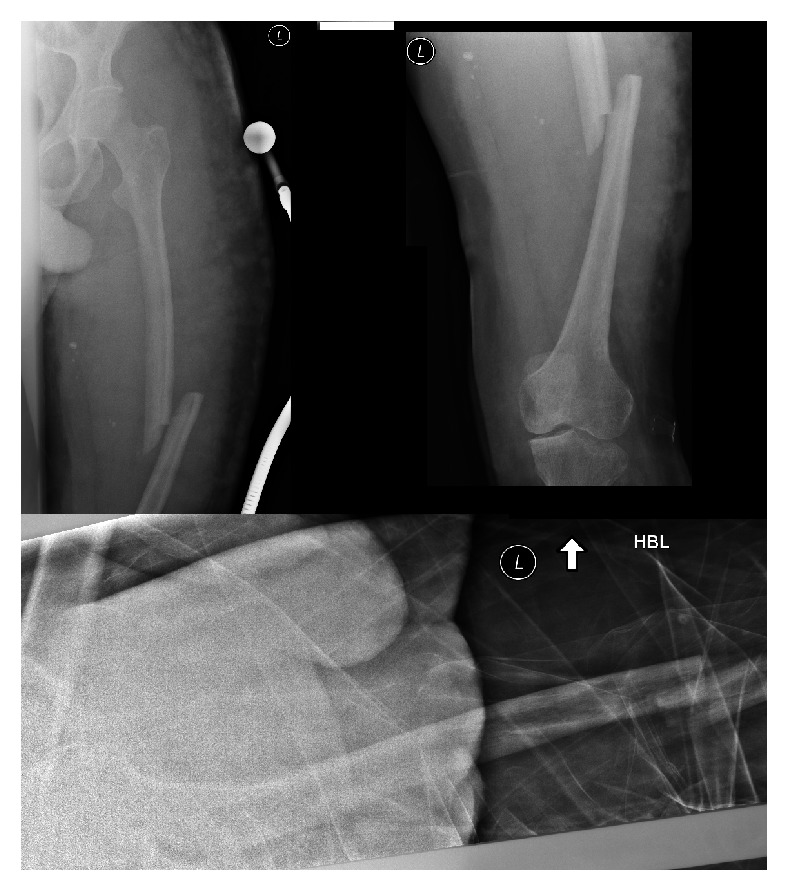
AP and lateral radiographs of the left femur, demonstrating a complete diaphyseal fracture.
